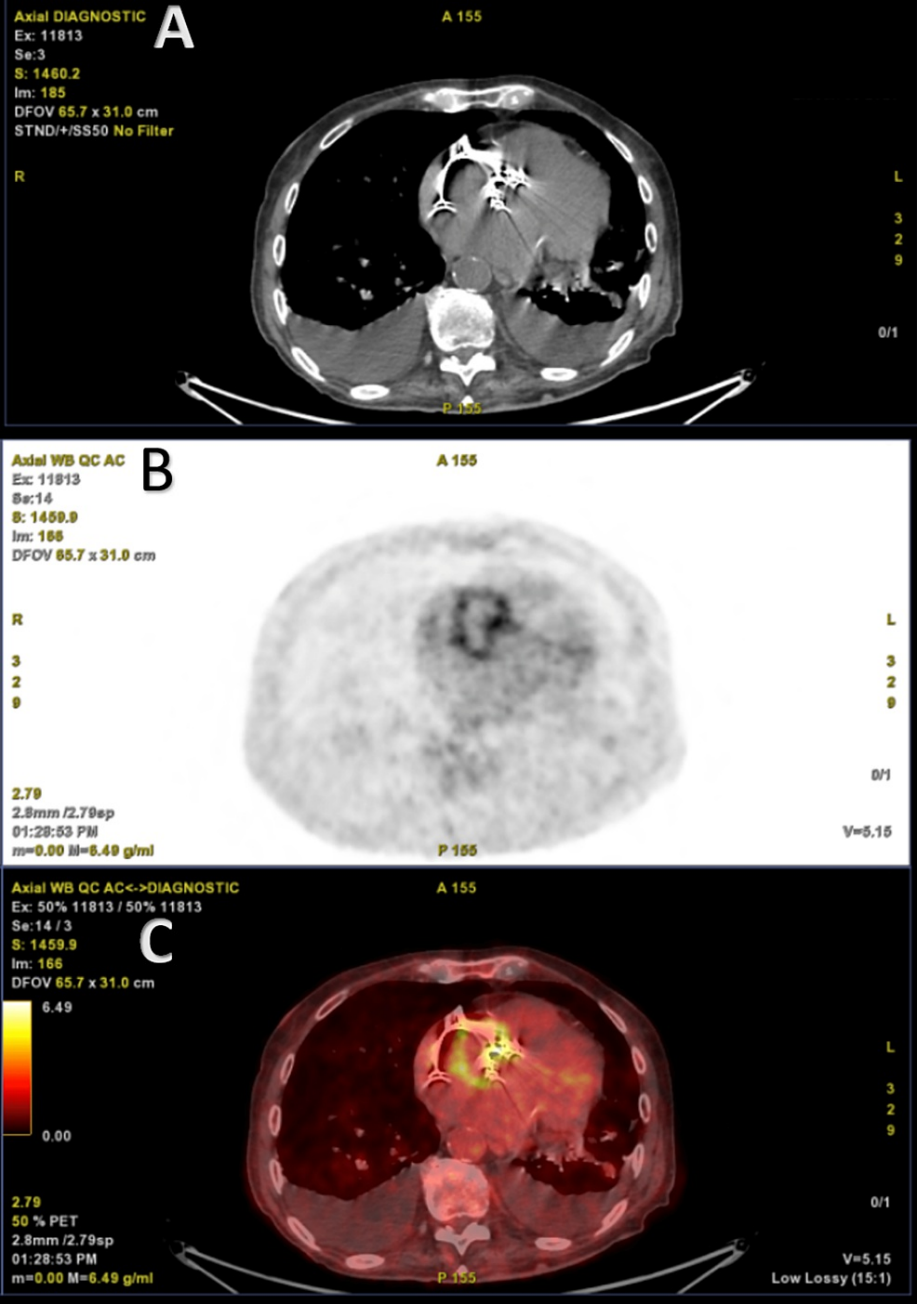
FDG positron emission tomography (PET) scan Transaxial CT scan (A), FDG-PET (B), and fused PET/CT (C) views. ­­There was an increased FDG uptake around the bioprosthetic aortic valve without the involvement of the pacemaker leads. FDG = fluorodeoxyglucose (18F).
